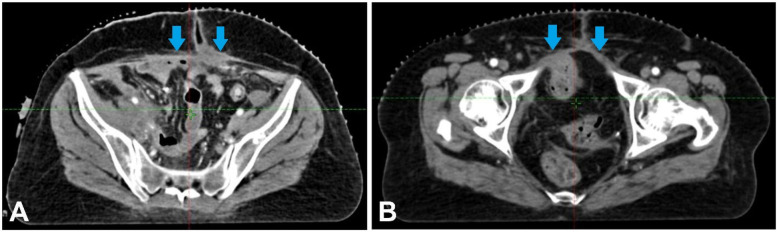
(A,B) Showing the post-surgery CT scan images with mild thickening involving the post-operative bed upto the scar site.
